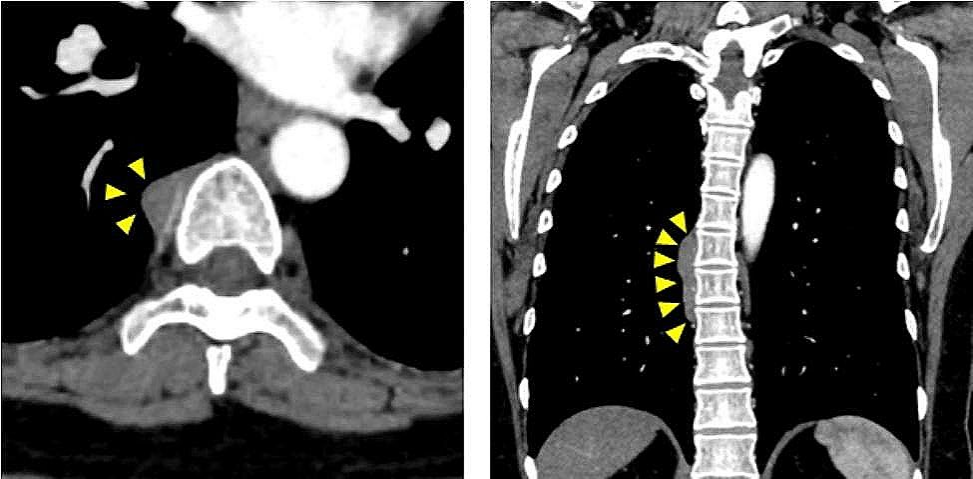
Thoracic contrast-enhanced computed tomography. Thoracic contrast-enhanced computed tomography reveals a mass, measuring 23 × 13 × 55 mm, on the right side of the seventh to ninth thoracic vertebrae. The area indicated by the yellow arrows shows the mass
